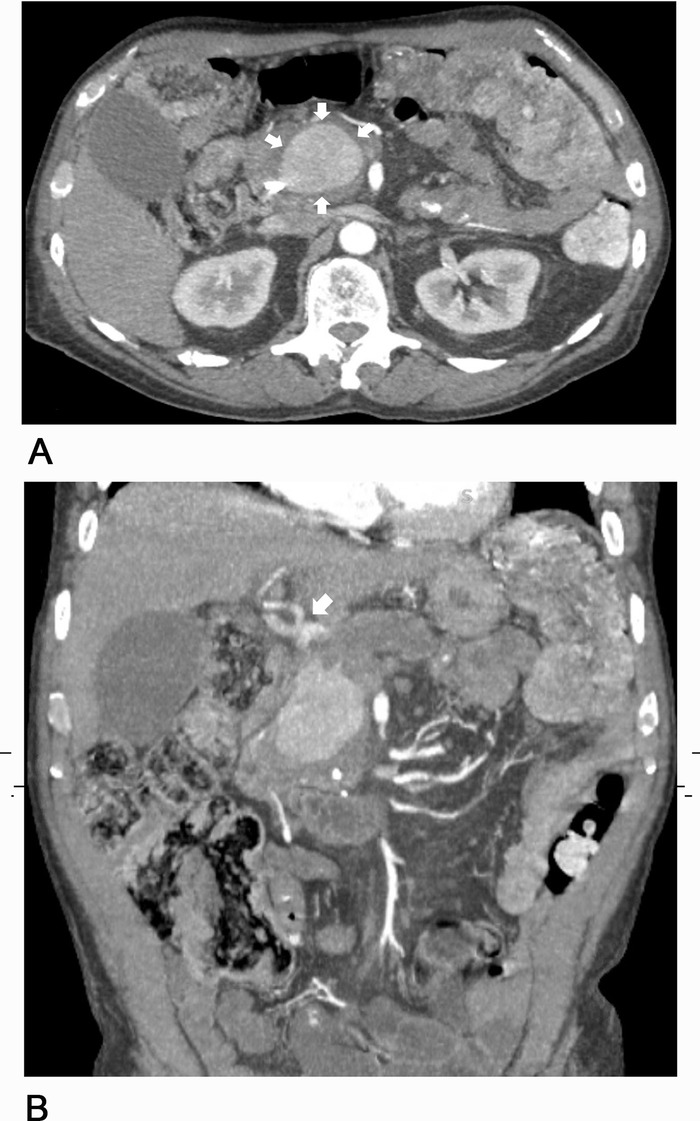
Computed tomography angiography showing (A) axial cut of gastroduodenal artery pseudoaneurysm measuring 3.8 × 4.9 × 5.4 cm (arrows) and (B) coronal cut with arrow indicating trifurcation of the common hepatic artery.
